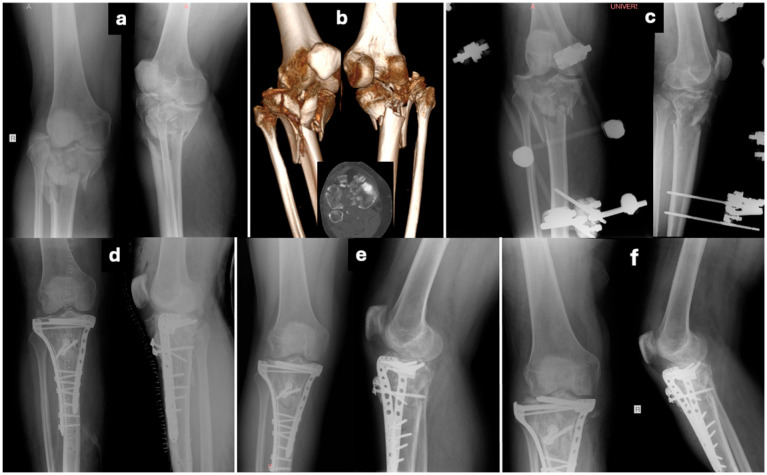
Pre-operative X-rays (a) of a 52-year-old male with a type-VI tibial plateau fracture according to the Schatzker classification, and anterior and posterior views of 3D reconstruction and axial CT scan (b). Post-operative X-rays after temporary trans-articular external fixation due to soft tissue contusion (c). Post-operative X-rays after ORIF of medial and lateral tibial condyle and tibial tubercle via a single anterior approach 2 weeks after injury (d). One-month and three-month follow-up anteroposterior and lateral X-rays, revealing progressive subsidence of the joint surface of the lateral tibial condyle (e,f).
